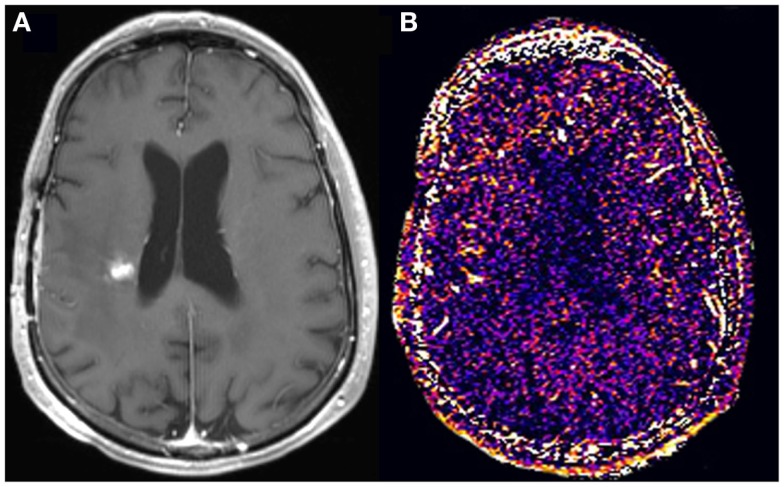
A new enhancing lesion appeared adjacent to the resection cavity 3 months following completion of chemoradiation (A), without evidence of elevated kTrans (B) on DCE-MRI. The lesion remained unchanged in size for the subsequent 4 months, consistent with pseudoprogression.
